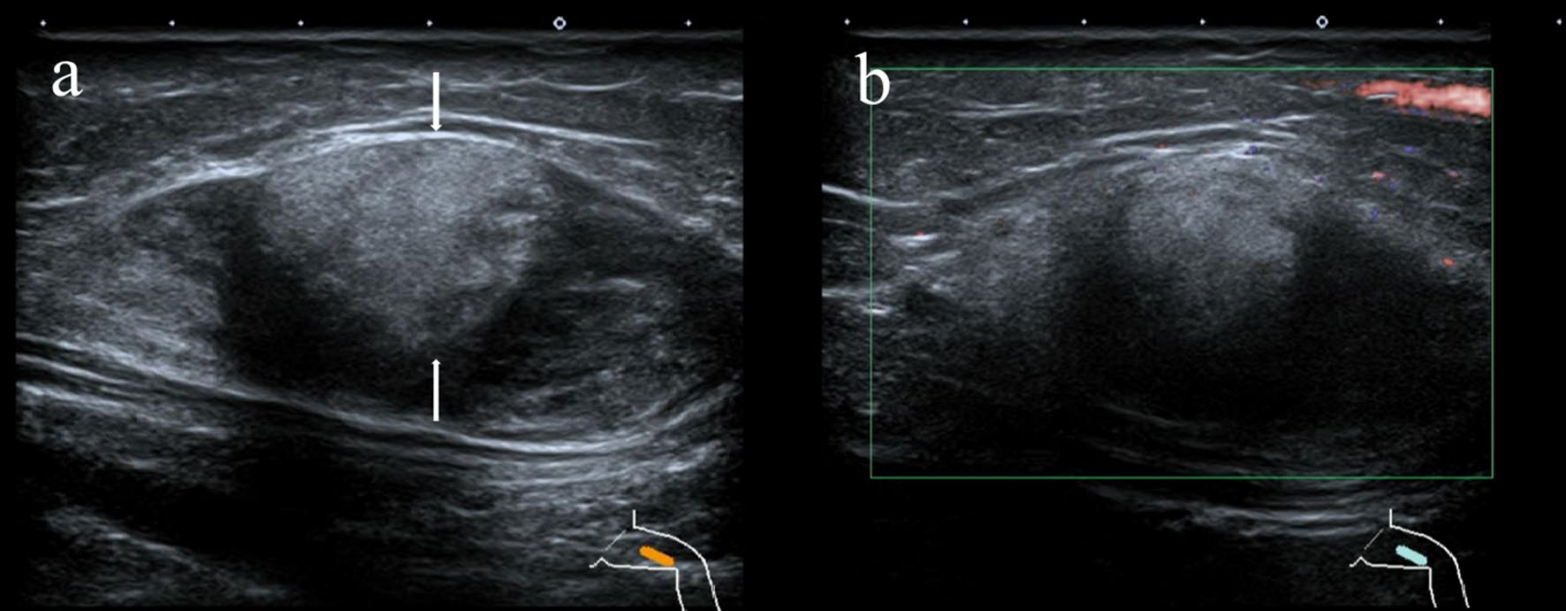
In a 4-year-old girl, an adipoblastoma was detected in her leg. (a) Gray-scale ultrasound examination showed a mixed echogenic, ill-defined mass lateral to the left sartorius muscle, with a patchy hyperechoic area (long arrow). The mass did not break through the deep fascia or invade the subcutaneous fat layer. (b) Color doppler flow imaging did not show an obvious blood flow signal within the mass.
